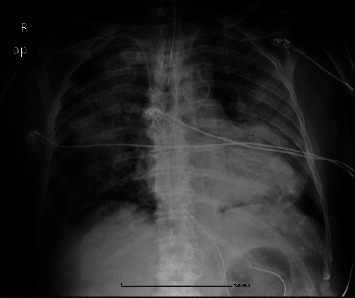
Chest X-ray (bedside) on the fourth day of hospitalization in the ICU after clinical deterioration depicting diffuse bilateral opacities.
